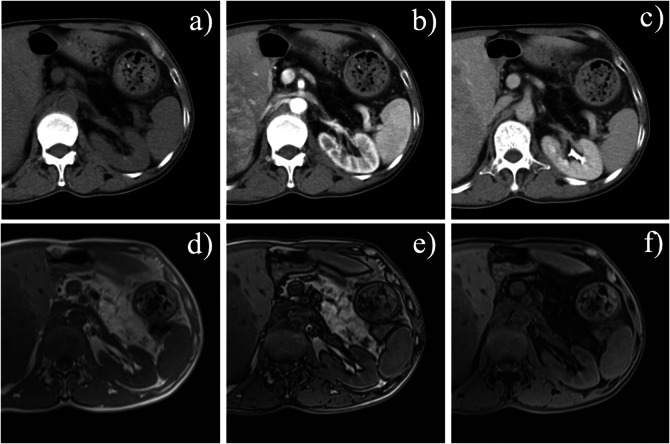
A 50-year-old man with intra-pancreatic fat deposition coexisted with hepatocellular carcinoma. a–c Axial unenhanced and contrast-enhanced computed tomography (CT) images showed fat density in the pancreatic area without enhancement. Axial in-phase (d) and out-of-phase (e) T1-weighted MR images showed hyperintensity signal in the pancreatic region, and this region was hypointense on T1-weighted fat-suppressed MR image (f). At the same time, part of the liver tumor can be seen in these images.
